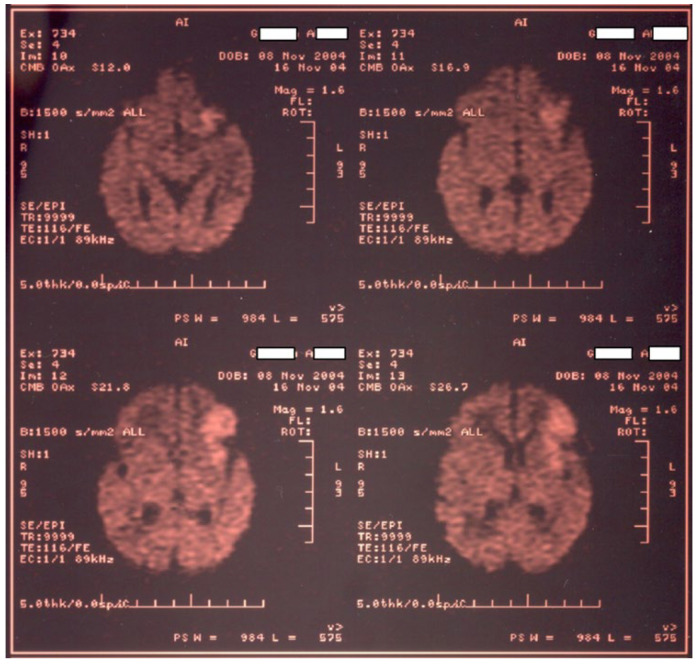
MRI examination for newborn #1 confirms a large right frontal–parietal infarction.
